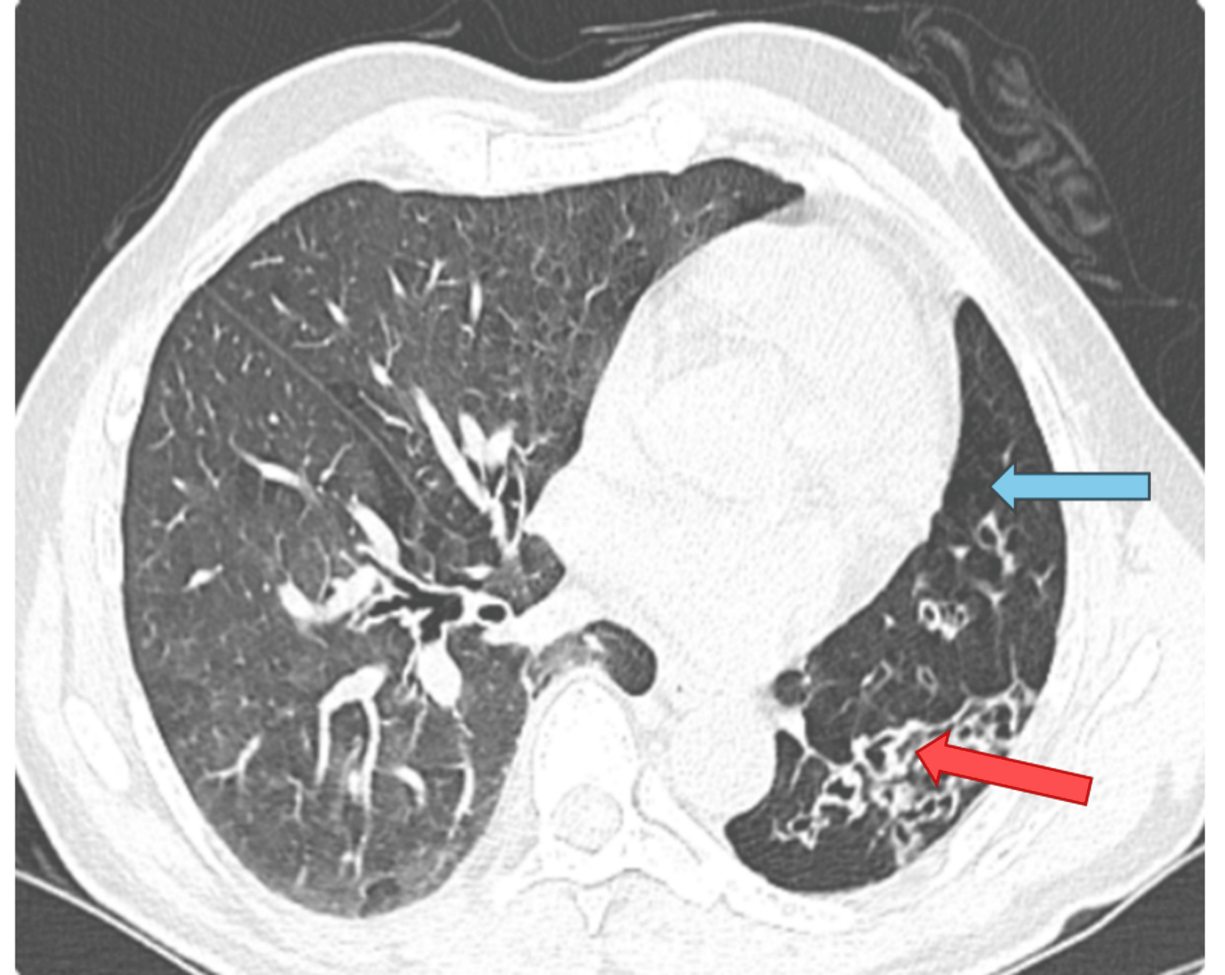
Axial-section CECT chest (lung window) shows diffuse hypoattenuation (blue arrow) of the left lung with left lower lobe cystic bronchiectasis (red arrow). CECT: Contrast-enhanced computed tomography
